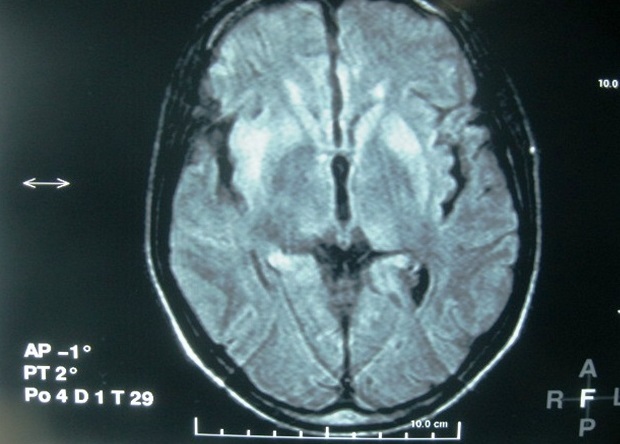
Brain MRI showing focal hyperintensity of the right sub-cortico-frontal region on FLAIR image
